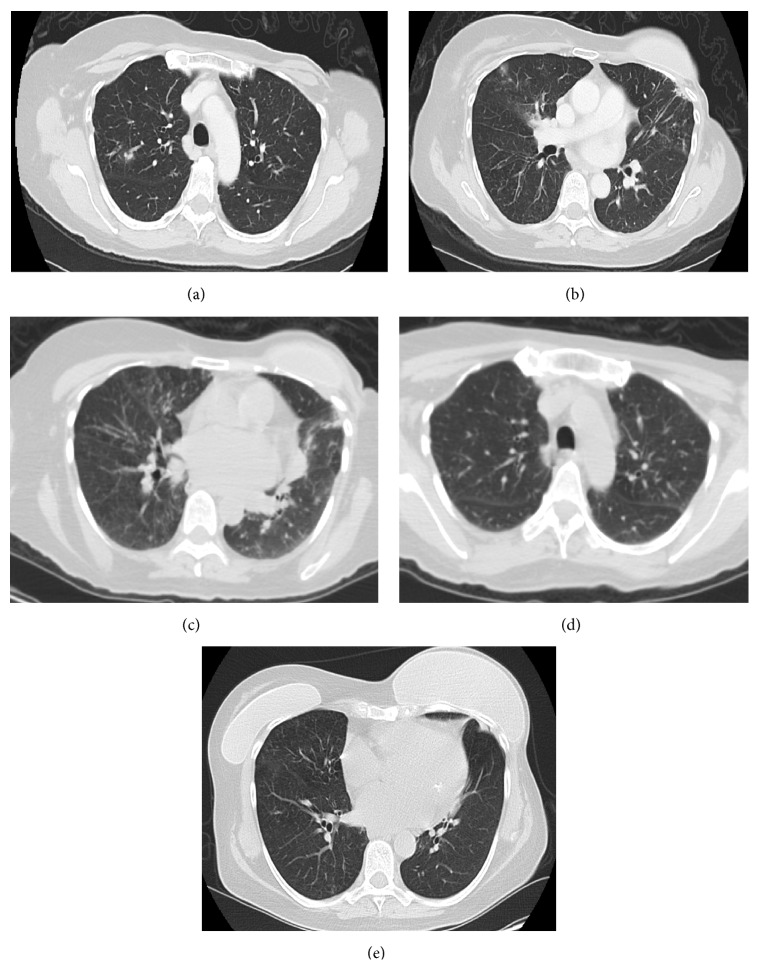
Chest CT findings in Case 2. Right upper and middle lobes nodular opacities (a) and lingular bronchiectasis (b). Waxing and waning opacities involving the right upper and middle lobes and lingula (c). Interval resolution of right middle lobe (d) and lingular opacities (e).
